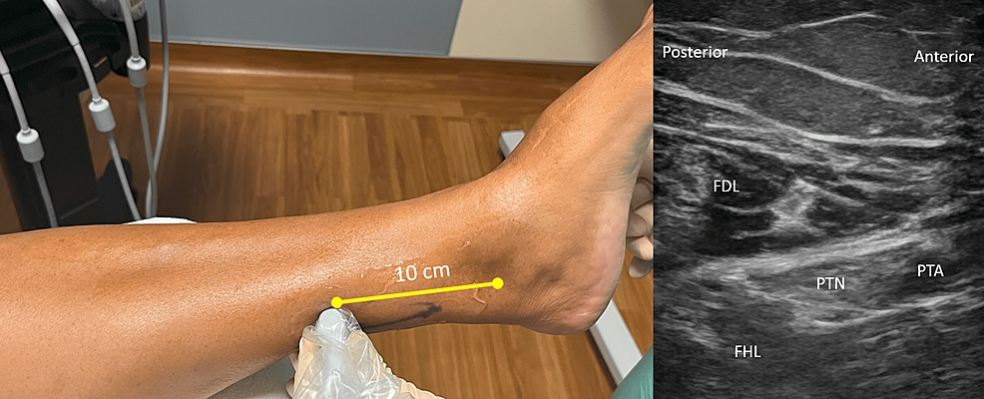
Placement of Ultrasound Probe and Labeled Sonoanatomy The ultrasound was placed 10 cm from the left medial malleolus. The sonoanatomy is labeled. FDL = Flexor digitorum longus, PTN = Posterior tibial nerve, PTA = Posterior tibial artery, FHL = Flexor hallucis longus, and cm = Centimeters.
